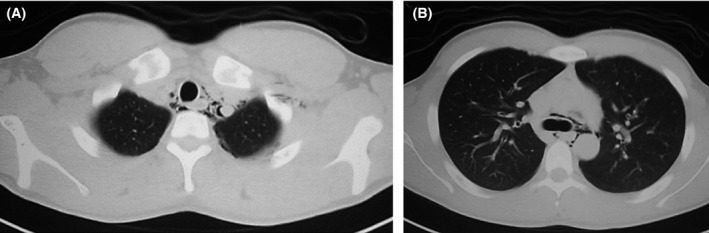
plain chest computed tomography showing pneumomediastinum.
